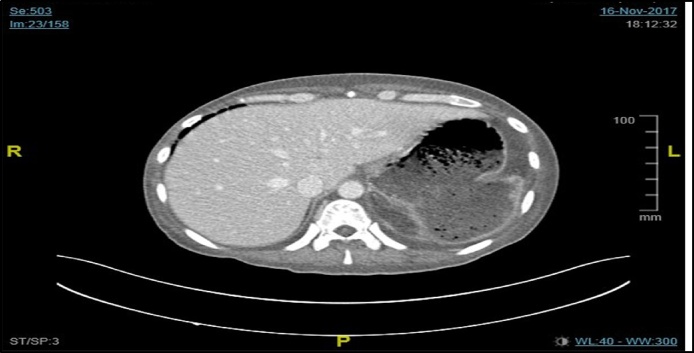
CT chest axial view showing splenic abscess invading the stomach.
